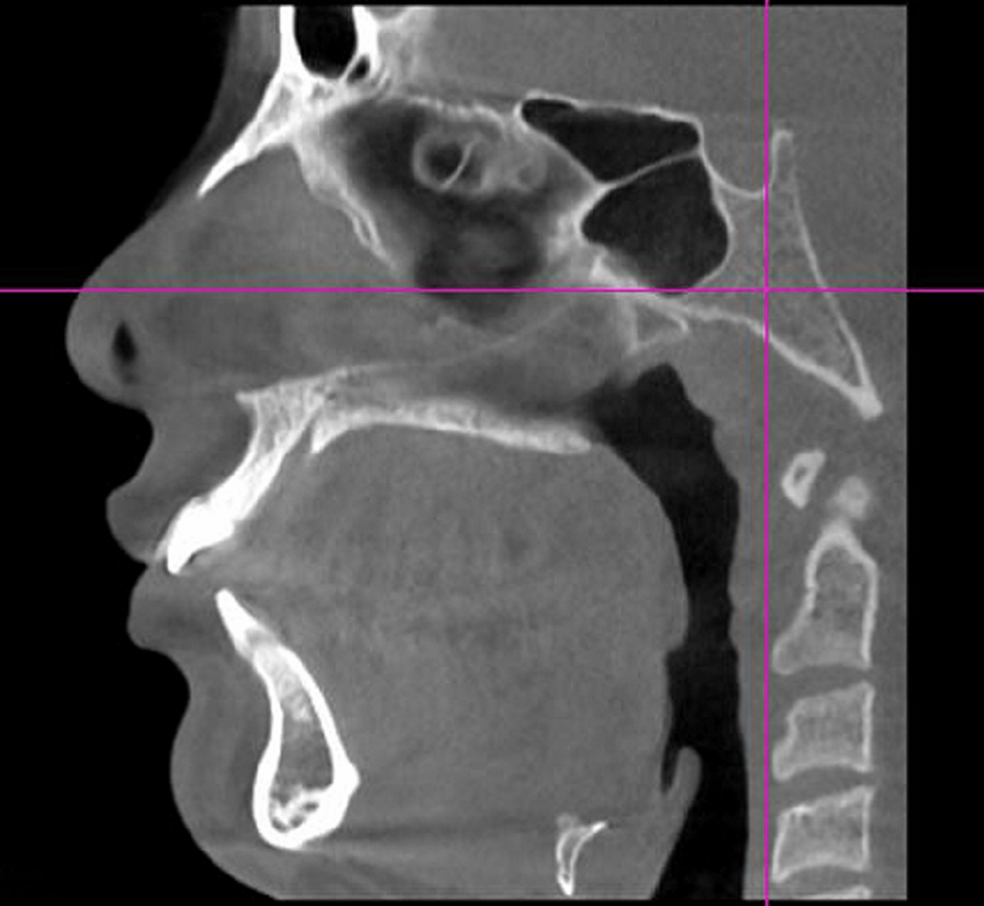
CBCT pretreatment with AdvanSync CBCT - Cone beam computed tomography
